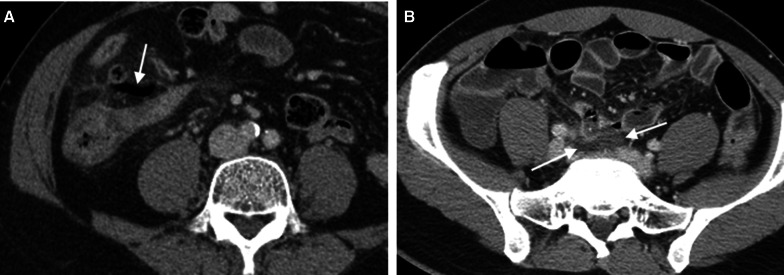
Periappendiceal air and fluid. Axial CT images of two different patients show extraluminal air within mesoappendix (arrow in a) and extraluminal periappendiceal fluid (arrow in b). The former patient is a 73-year-old man who presents with abdominal pain and fever, slightly elevated white blood cell counts (11,370 cells/mm^3^) and neutrophilia (88.0% neutrophils). The latter is a 39-year-old-man presenting with right lower abdominal pain for 1 day, elevated white blood cell counts (17,100 cells/mm^3^) and neutrophilia (86.8% neutrophils). Both have a confirmed diagnosis of perforated appendicitis
